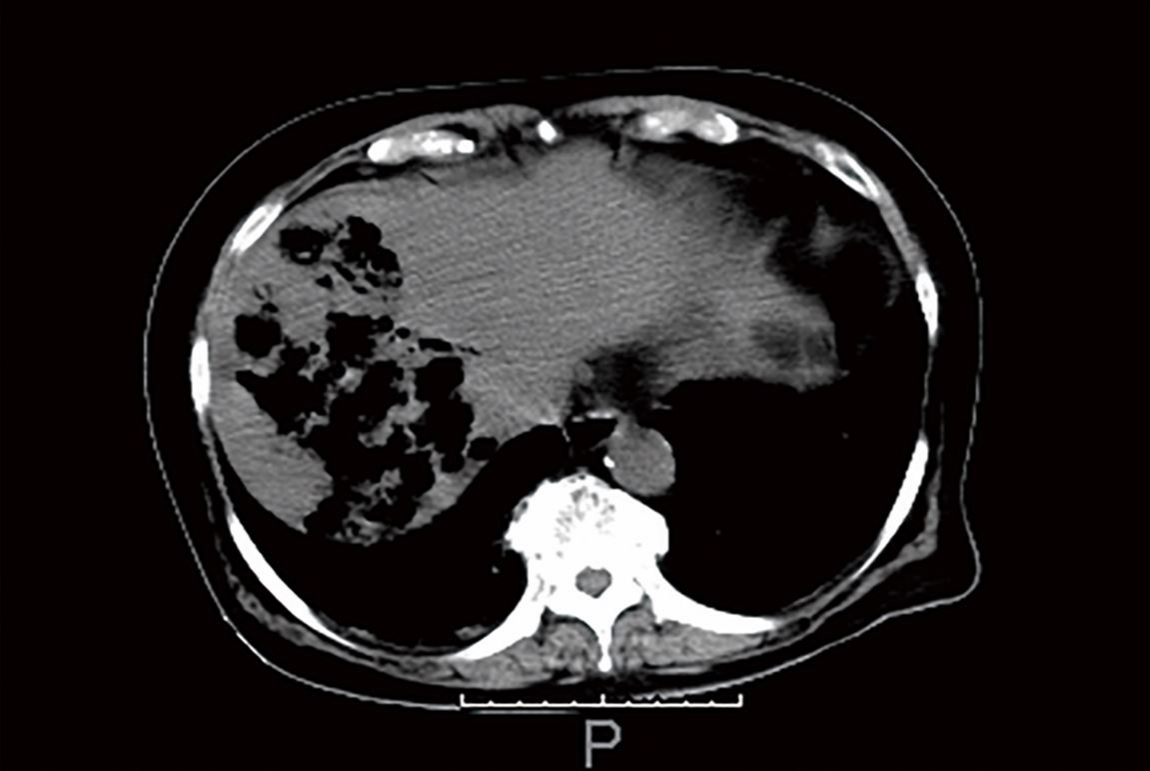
Non-contrast computed tomography of the abdomen. Irregular-shaped cavities containing abundant gas in the right lobe of the liver.
